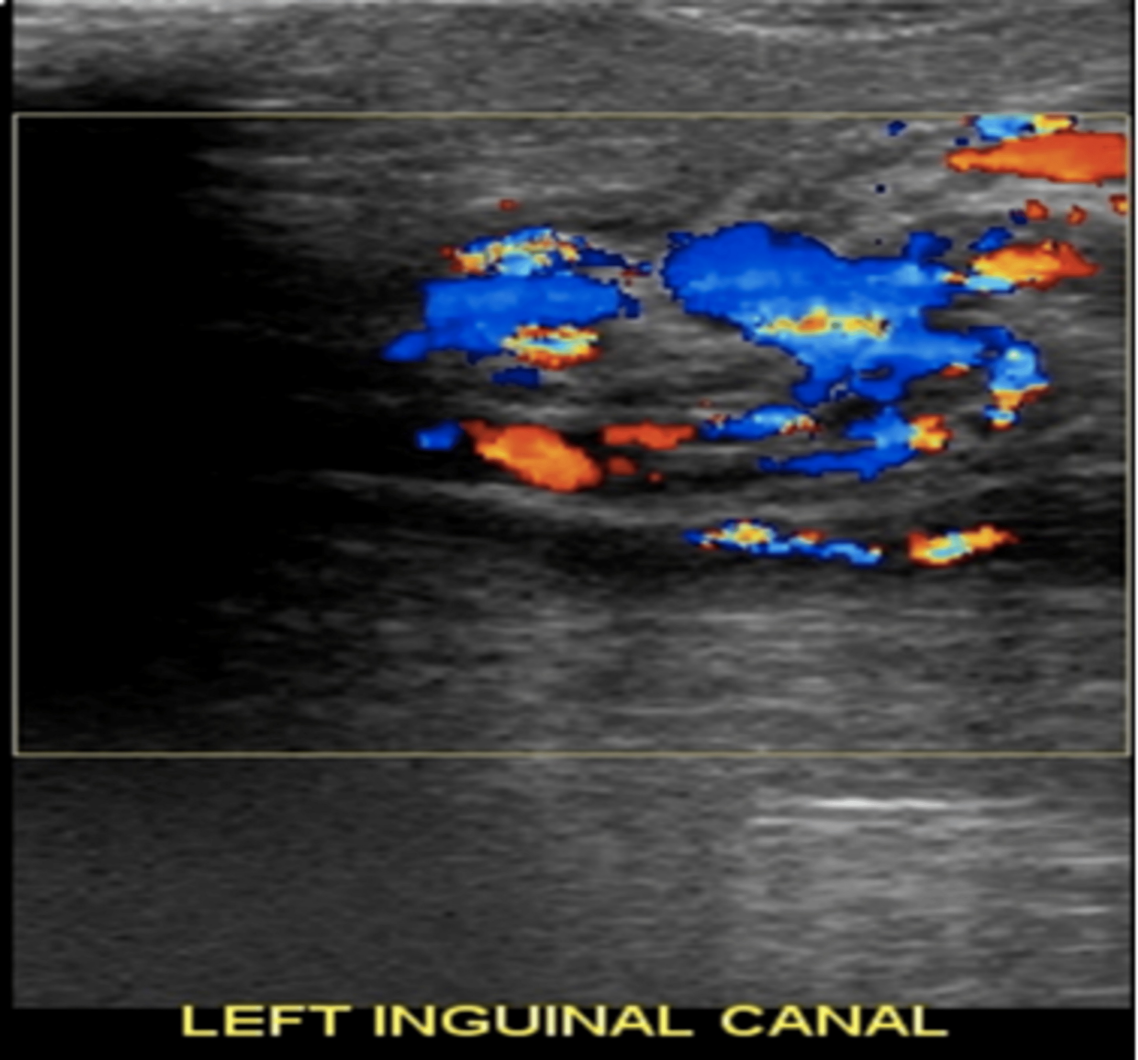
"Torsion knot sign" on color Doppler US of torsed vessels in the inguinal canal.
